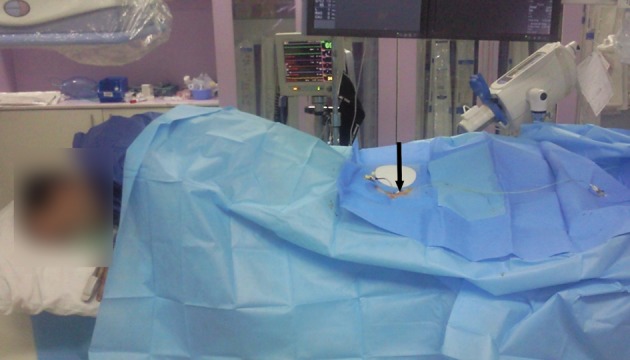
Figure 1. A 41-year-old man, known case of ankylosing spondylitis, semi-prone on the angiographic table. Under sonographic guide a 6 french sheet was inserted in his left thrombosed popliteal vein (arrow)
